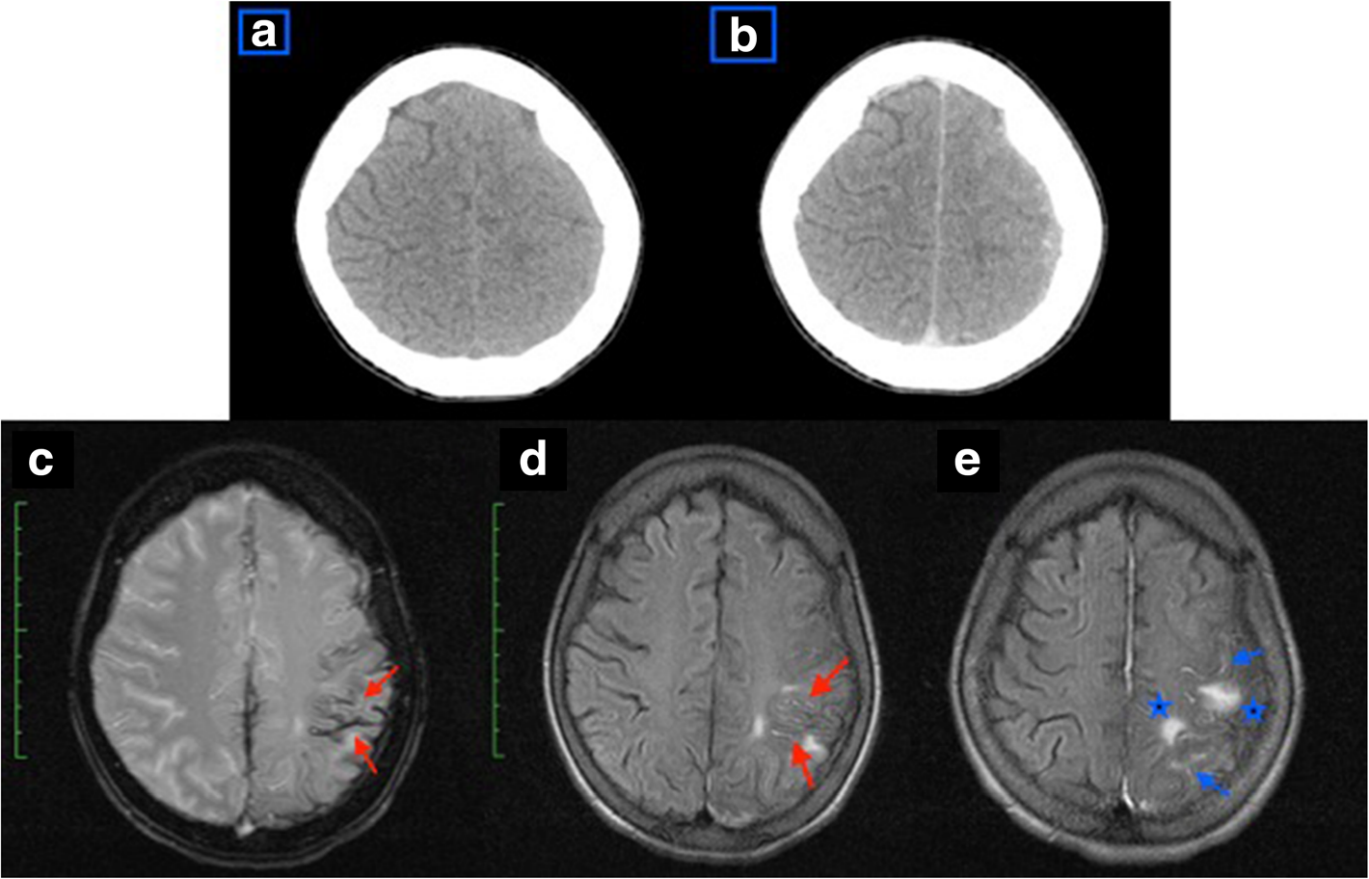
Axial CT images at the high parietal region, plain (1a) and contrasted (1b) showing; 1a – left high parietal region cerebral edema is present, with no evidence of haemorrhage. 1b – Contrast is seen opacifying the sulci, but otherwise no additional findings were evident. Axial MRI images; GRE (1c), pre-contrast FLAIR (1d), and T2 FLAIR post gadolinium (1e), sequences at the high parietal region showing; 1c – Hypointense signal of the affected region (arrows) with corresponding hyperintensity on FLAIR (1d) confirming acute convexal SAH. 1e – Areas of pachy- and leptomeningeal enhancement, with small foci of haemorrhage; indirect signs of vasculitis
